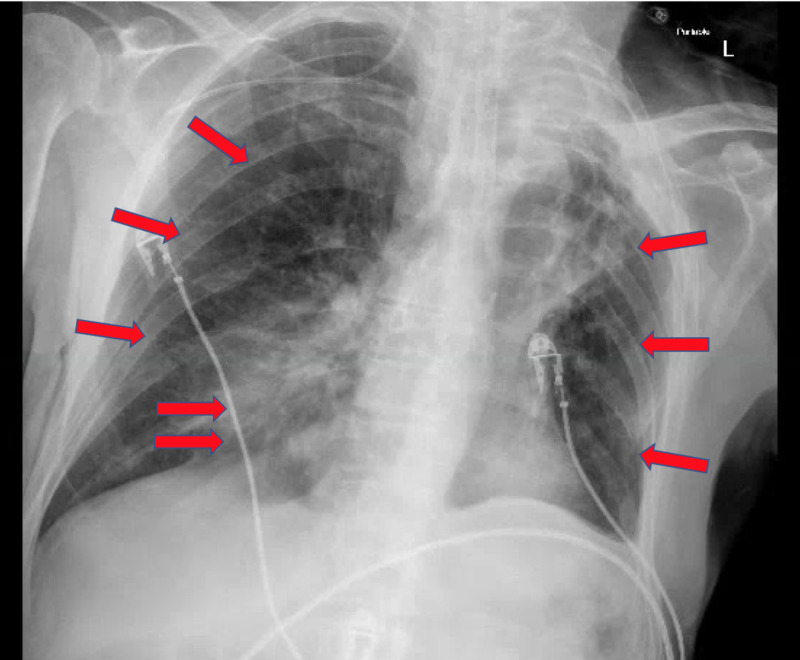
Initial chest x-ray on Day 1 of hospital admission shows bilateral patchy opacities secondary to severe acute respiratory syndrome coronavirus 2 (SARS-CoV-2) viral pneumonia and right lower lobe consolidation secondary to bacterial superinfection
